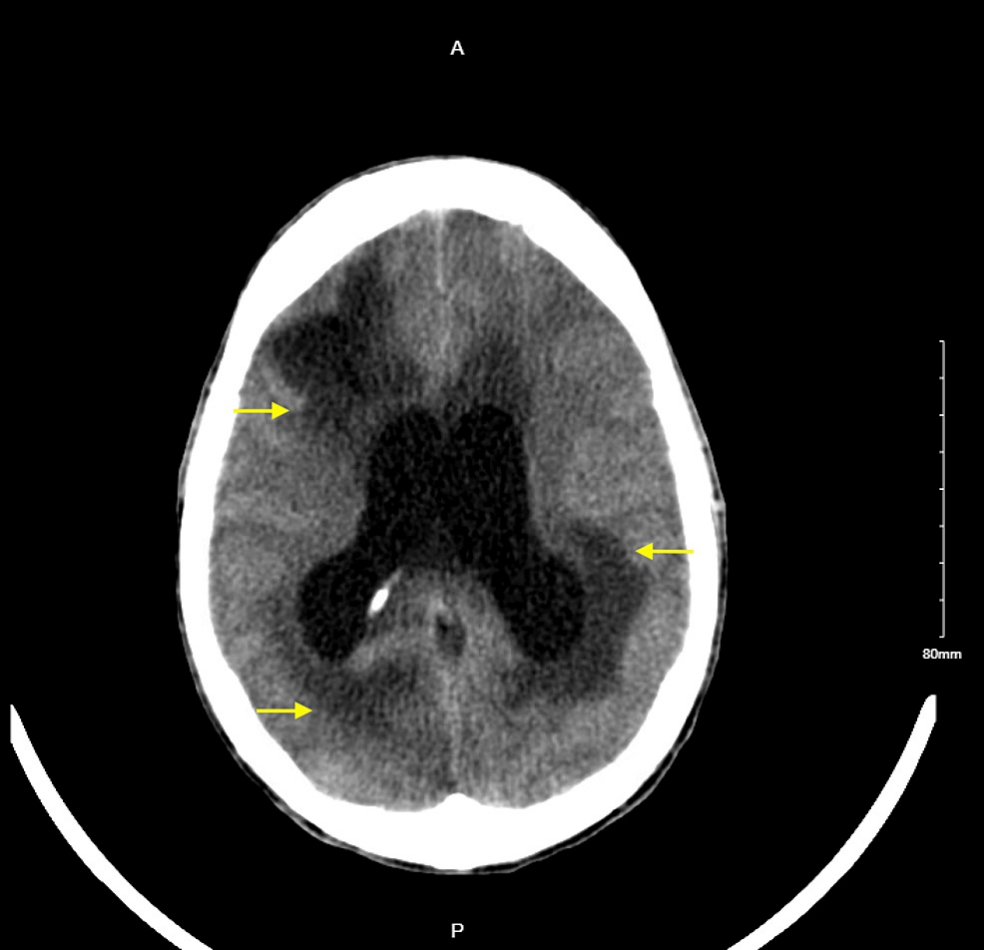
CT head obtained due to worsening mental status four days after the removal of the external ventricular drain: diffusely accentuated gray-white differentiation most compatible with diffuse/global vasogenic edema, as well as some new edema (presumably vasogenic) surrounding the right transfrontal ventricular shunt/catheter tract (yellow arrows).
